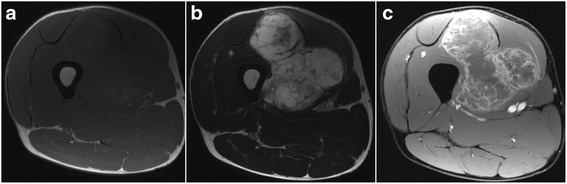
Magnetic resonance imaging findings. a T1-weighted axial imaging reveals a soft tissue tumor with an isointense signal. b T2-weighted axial imaging reveals a soft tissue tumor with a high-intensity signal. c Axial imaging with gadolinium enhancement at the side edge of the partition structure
